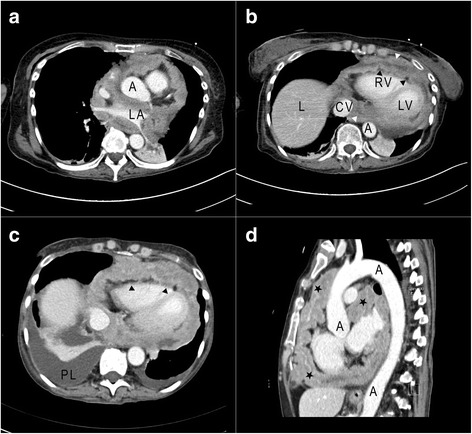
Contrast-enhanced computed tomography of the thorax before (a, b) and after (c, d) chemotherapy. a Axial image crossing the left atrium. b-c Axial images through the ventricles. d Sagittal image through the aortic arch. Before chemotherapy (a, b), both parietal (*white arrows*) and visceral (*black arrow*) layers of the pericardium were very thickened. After chemotherapy (c, d), computed tomography shows progression of the lesions around ventricles, in cranial sinuses, around the ascending aorta and the pulmonary artery. *Legend:*
